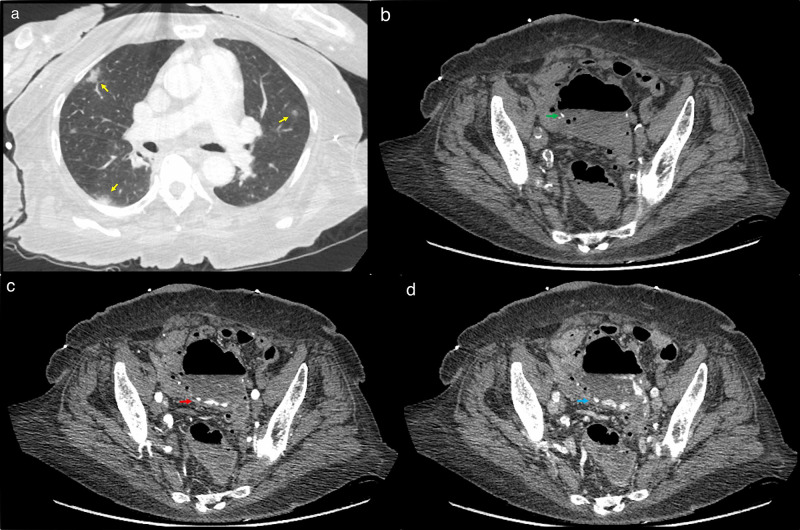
Radiological findings in patient 5 Axial image from CT angiogram of the abdomen and pelvis (a) at the lung bases shows peripheral ground-glass opacities bilaterally (yellow arrows). Axial image in (b) non-contrast phase shows an ileosigmoid anastomosis (green arrow), with (c) intravenous contrast extravasation in the arterial phase (red arrow) and (d) pooling (blue arrow) in the sigmoid bowel lumen distal to the anastomosis on venous phase to suggest active gastrointestinal bleeding. The source appears to arise from an inferior mesenteric artery branch CT: computed tomography
